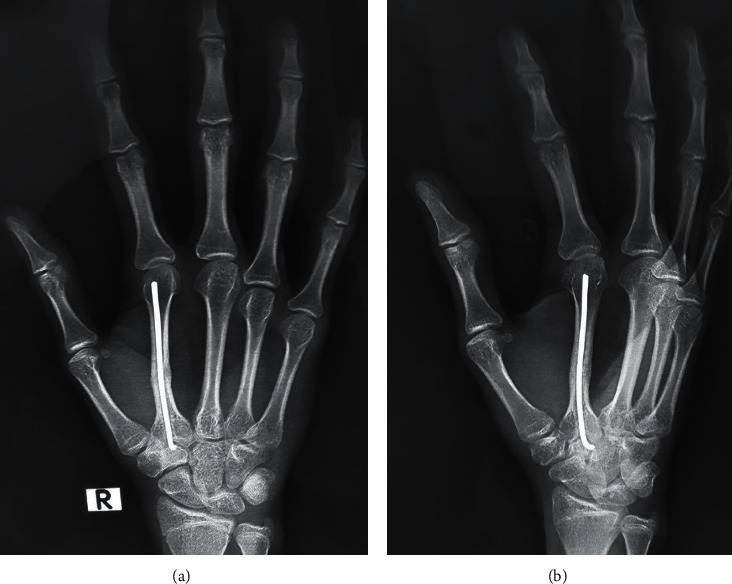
X-ray showing the 6-month follow-up of the patient, prior to K-wire removal.
